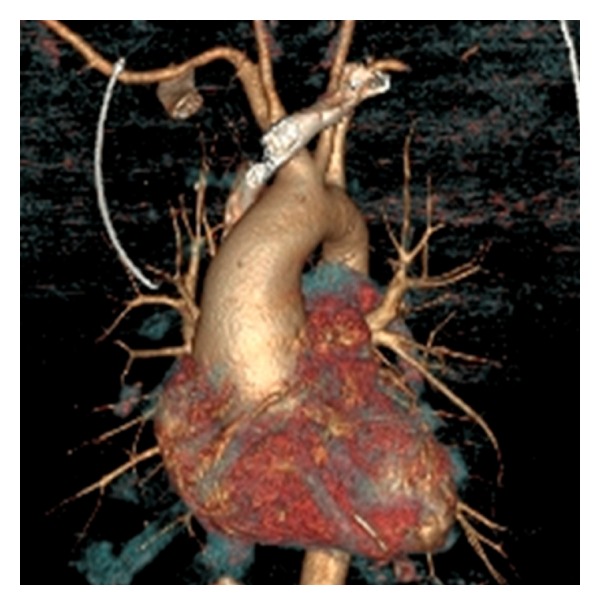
Computed tomography of a patient with a bicuspid aortic valve and aneurysmal dilatation of the aortic root, ascending aorta, and transverse aortic arch (Stanford cluster IV).
